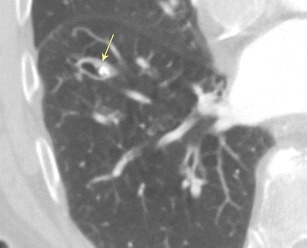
Bronchial obstruction due to a broncholith. CT image demonstrating calcified material (*arrow*) within the right lower lobe bronchus
